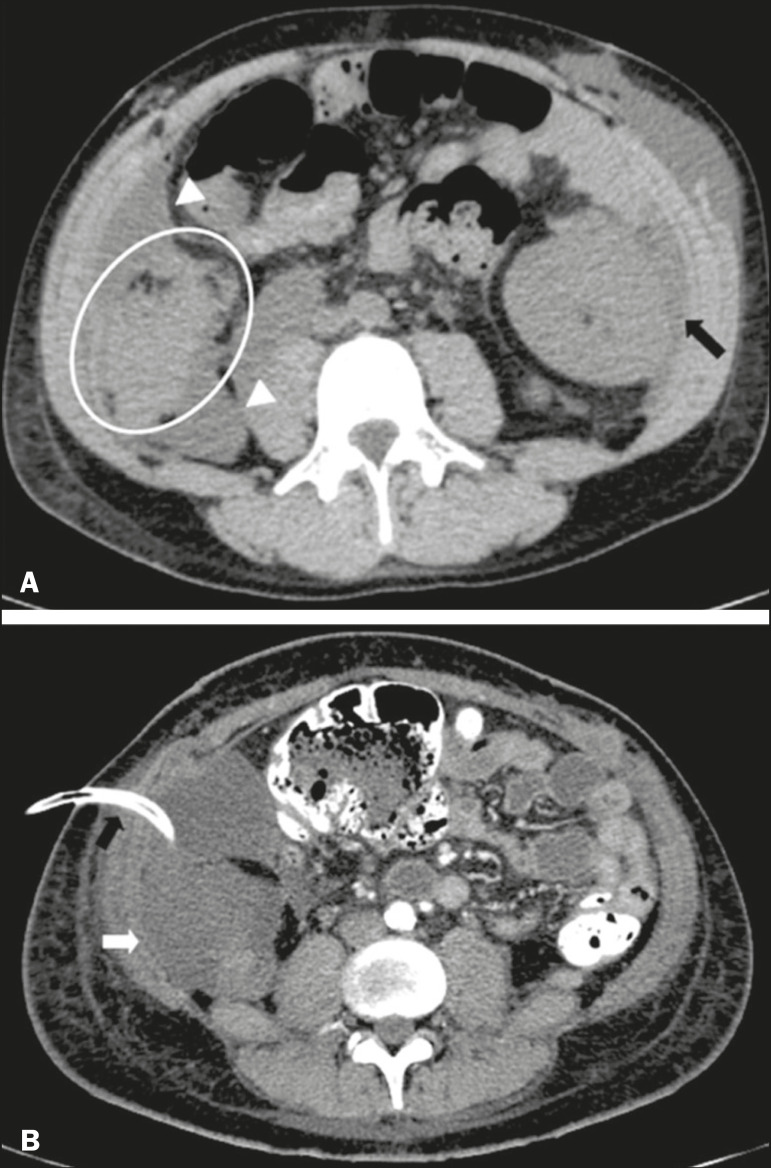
A: Unenhanced axial CT scan of the abdomen, showing fluid collections (arrowheads) adjacent to the pancreatic graft (ellipse). Note also the minimal amount of liquid adjacent to the renal graft on the left (black arrow). B: Fluid collection (white arrow) in the right iliac fossa of patient who underwent SPKT with percutaneous drainage (black arrow) seen on an axial CT scan of the abdomen with oral contrast.
